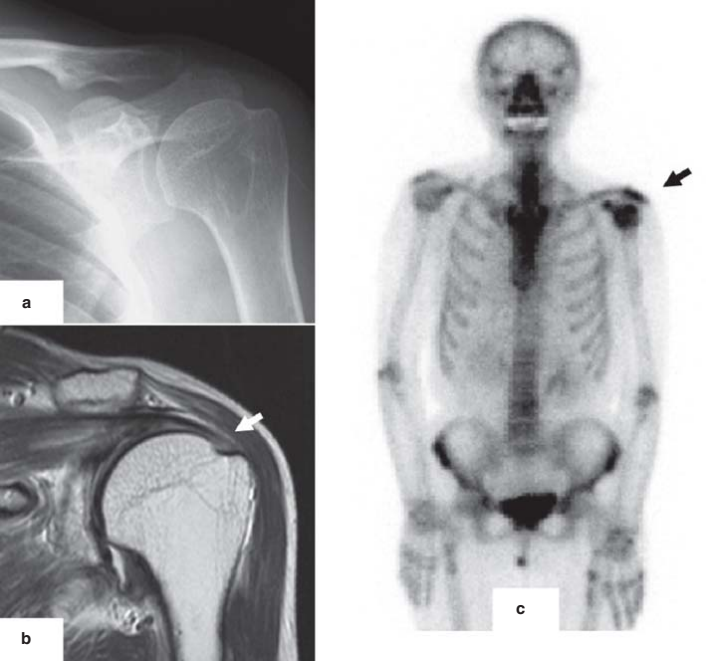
Case 1: A 71-year-old male with a rotator cuff tear in the left shoulder. No obvious changes of osteoarthritis are observed on plain X-rays (A). A full-thickness rotator cuff tear is observed on the coronal T2 WI MRI (B, arrow). An increase of RI uptake in the left shoulder joint is observed in the bone scintigram (C, arrow). However, there are no left–right differences in RI uptake for elbow or wrist joints.
